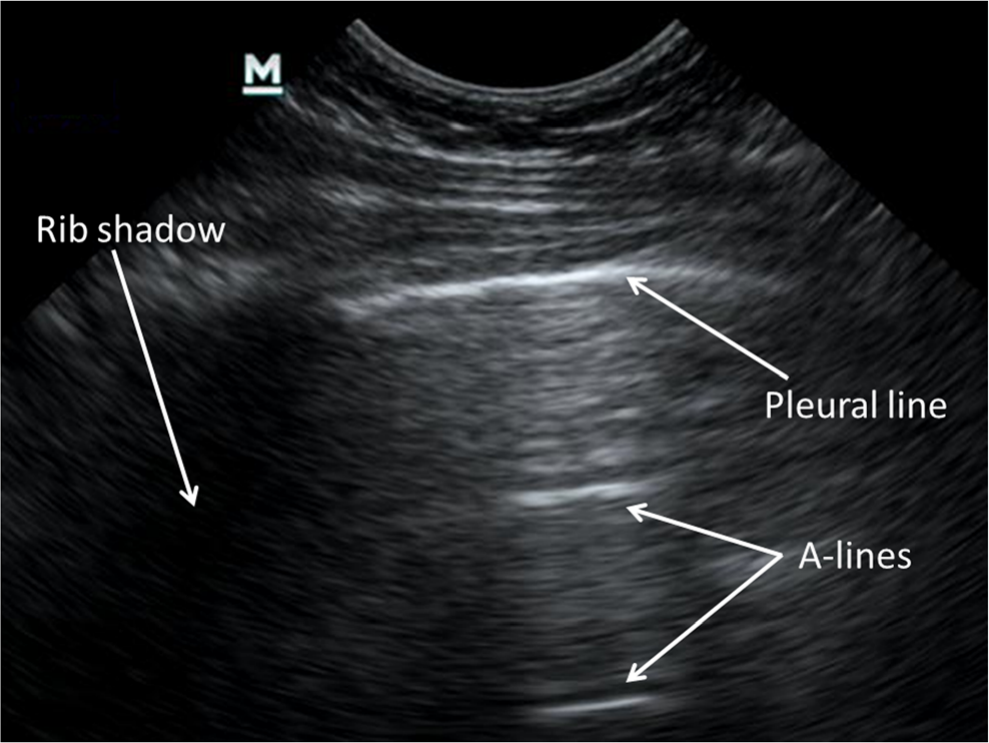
An ultrasound image from the right anterior upper lung zone in a 16-month-old girl demonstrates normal lung echo pattern with a smooth, hyperechoic pleural line, A-lines and no B-lines
